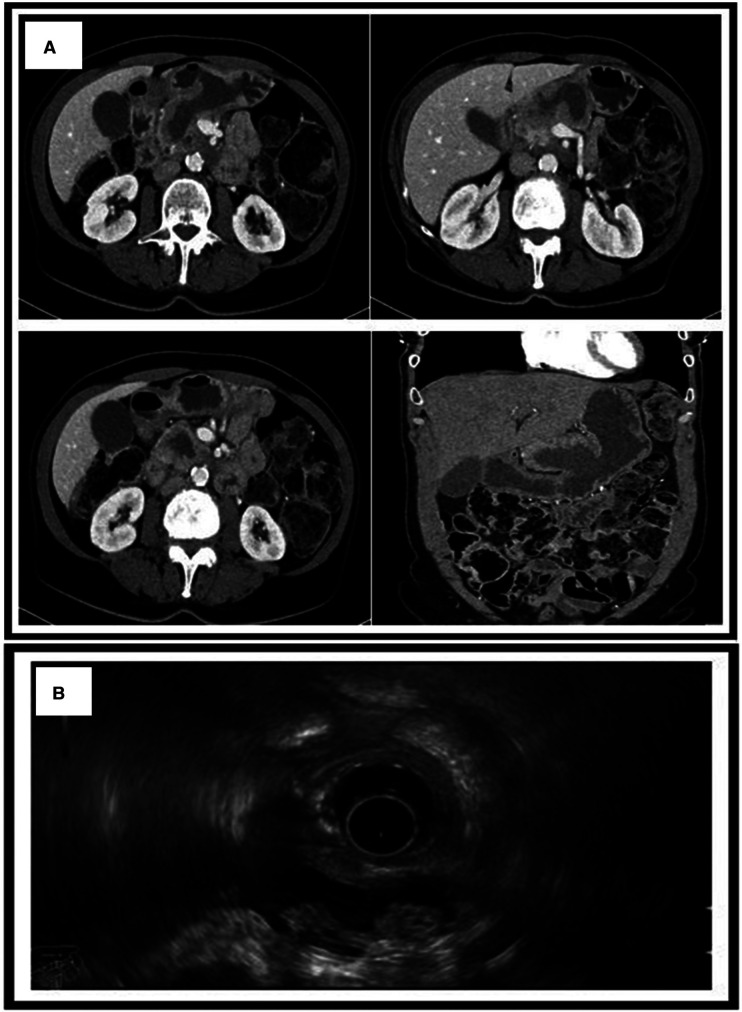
(A) CT scan showing the MPD dilated to 1.3 cm with the presence of a fistula between the main pancreatic duct and the stomach. (B) Endoscopic ultrasound showing a non-homogenous pancreas with diffused calcifications with a dilated MPD of 1.3 cm. MPD, main pancreatic duct.
